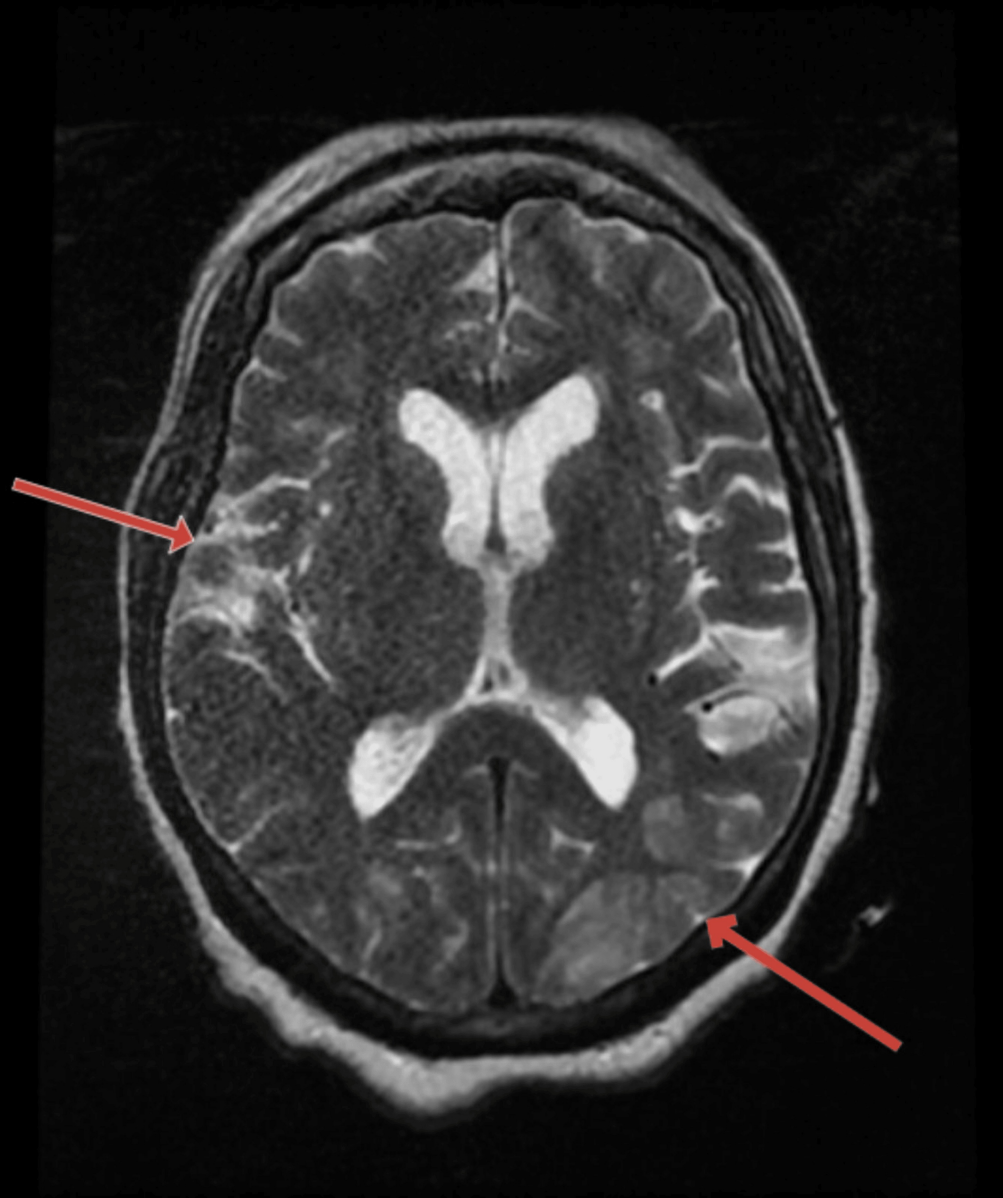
MRI Brain Showing Confluent T2 FLAIR Hyperintensity in the Bilateral Parietal and Occipital Lobes (Red Arrows) FLAIR: fluid attenuated inversion recovery
